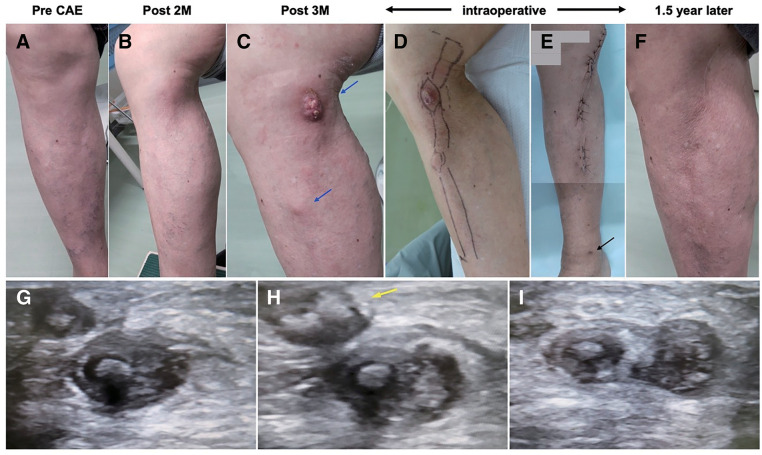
Preoperative clinical and ultrasound findings of the right lower leg. Preoperative clinical courses are shown in (A–F), and the ultrasound findings are shown in (G–I). (A) Before CAE. (B, C) Slight phlebitis in the right lower leg along the glue-occluded GSV 2 months after CAE. The phlebitis lesion that advanced to the venous abscess (C; blue arrows) and the lesion in the knee joint created a skin fistula (C) at 3 months postoperatively. (D, E) Preoperative and postoperative macroscopic findings. (E) The puncture site at the time of CAE at the right GSV around the medial malleolus (black arrow). (F) Infective phlebitis recurrence was not observed at 1.5 years after removal of the GSV. (G) The GSV is filled with a thrombus containing cyanoacrylate glue, and the vessel wall is edematous. Venous abscess is observed at the occluded GSV (H, I) along with fistula formation and a subcutaneous abscess (yellow arrow) at the puncture site. CAE: cyanoacrylate glue embolization; GSV: great saphenous vein
